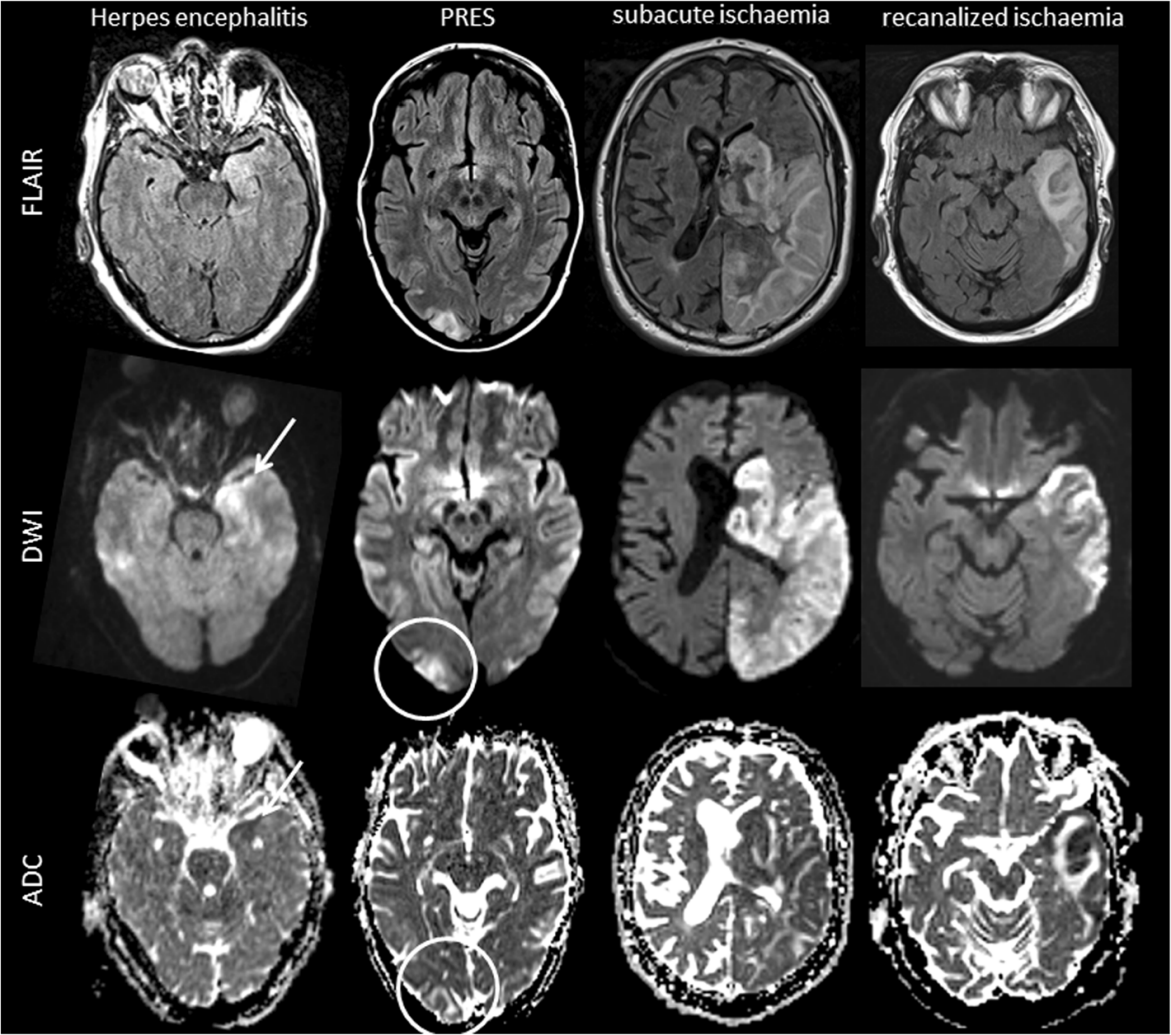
Imaging findings in MELAS differential diagnoses. Exemplary FLAIR, DWI and ADC images of diseases that can mimic MELAS lesions on MRI including herpes simplex encephalitis, posterior reversible encephalopathy syndrome (PRES), subacute territorial ischemic stroke and recanalized territorial ischemic stroke are displayed. Herpes simplex encephalitis may mimic many MELAS MRI findings including cortical restricted diffusion (arrow), subcortical vasogenic oedema and local mass effect on FLAIR images. Nevertheless, herpes simplex encephalitis lesions usually affect mesiotemporal areas and spread continuously. PRES lesions are typically located in the occipital and temporal lobes showing subcortical vasogenic oedema (circle). Subacute ischemic stroke is hyperintense on FLAIR and diffusion is restricted, but the lesion is confined within a vascular territory. In case of early recanalization, restriction of diffusion may be limited to cortical areas but is usually more pronounced than in MELAS
